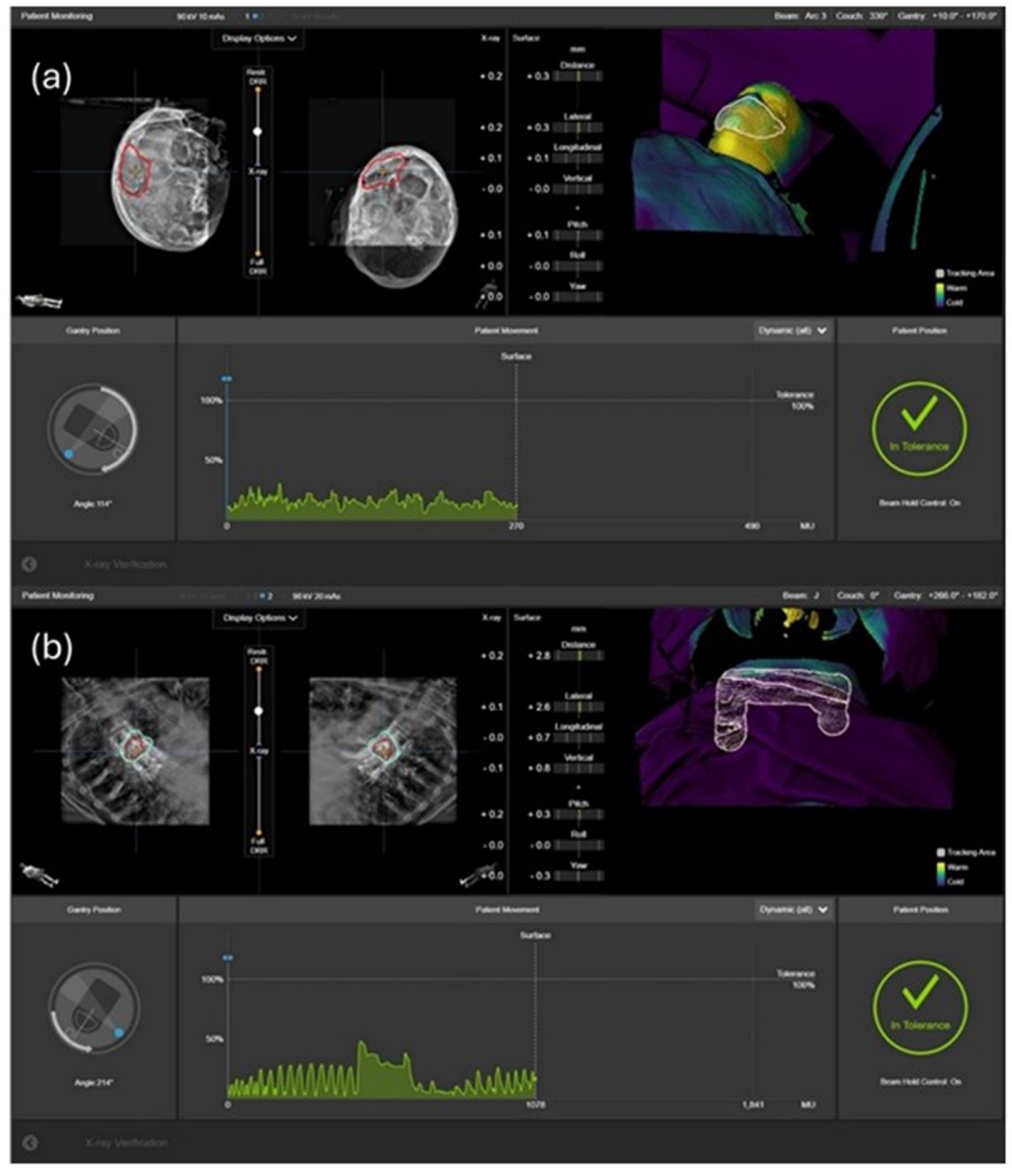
Real-time surface motion tracking alongside the pre-beam verification stereoscopic X-ray images for a cranial (a) and a spinal (b) case.
